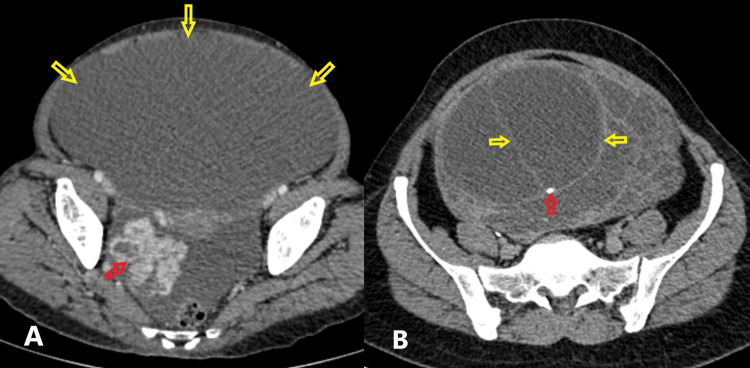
Contrast-Enhanced Computed Tomography (CECT) images A) CECT abdomen showing large showing large solid-cystic mass. The cystic component is marked with yellow arrows and solid component is marked with a red arrow; B) The mass has internal septations (marked with yellow arrows) and focal areas of calcification (marked with a red arrow).
